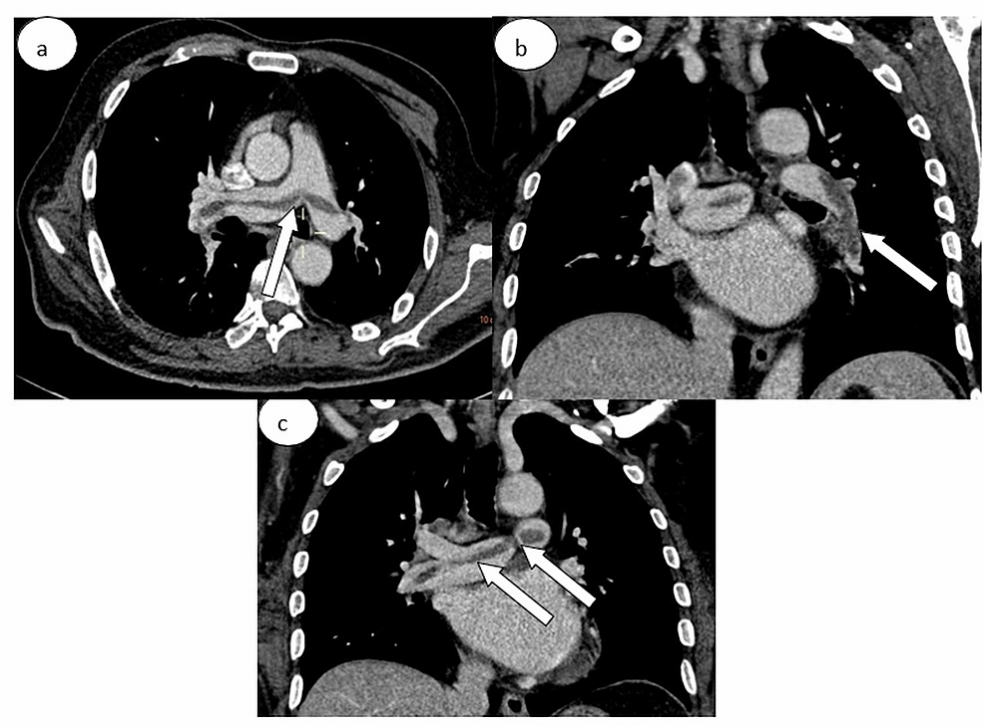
Computed tomography pulmonary angiography in (a) axial and (b, c) coronal windows showing the “railway sign” of acute pulmonary embolism (central filling defect surrounded by parallel lines of contrast) (white arrows) of the two pulmonary arteries, expanded to the left lower lobar branch
